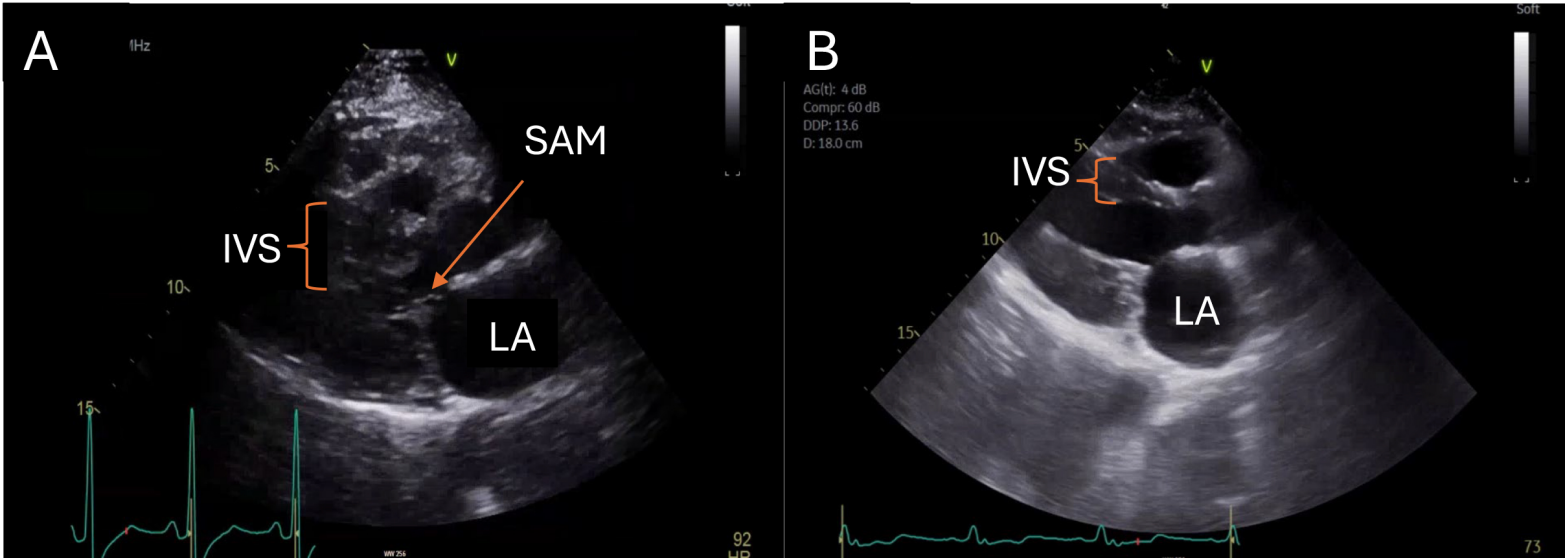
Parasternal long-axis views of a patient pre- and post-myectomy
